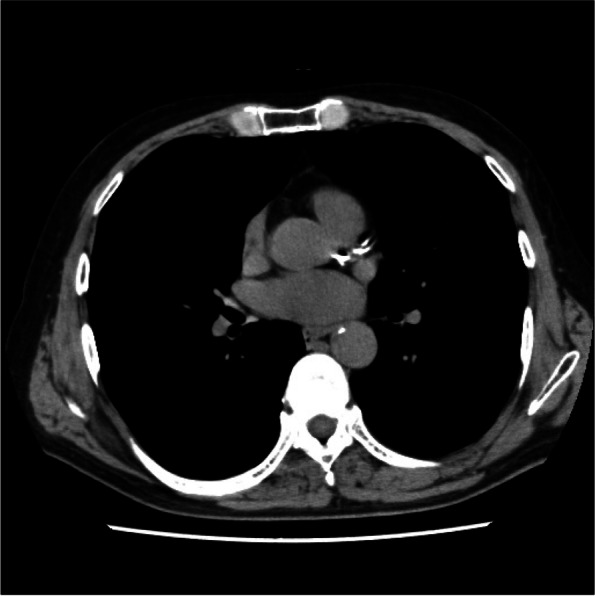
Computed tomography showed highly calcified coronary artery
